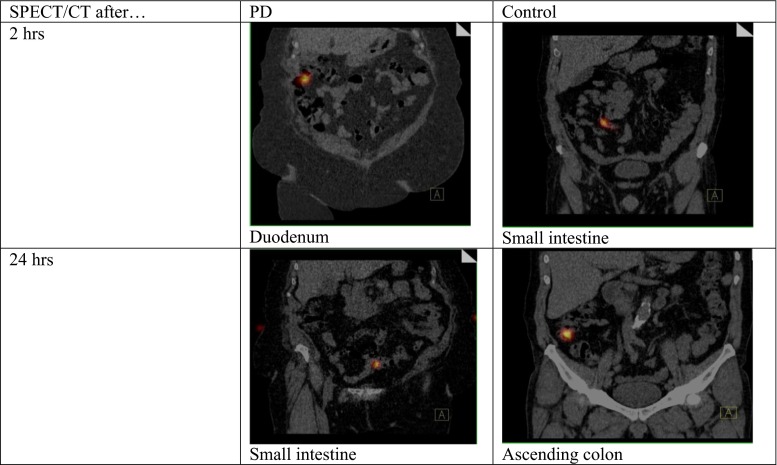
Typical picture of the fusion of SPECT and CT in PD and control subjects after 2 and 24 h after the intake of the capsule containing the isotope
